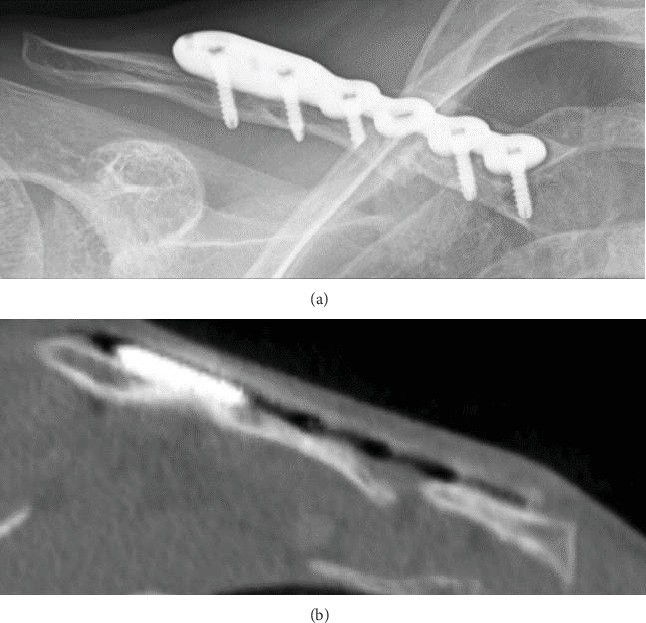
(a, b) Radiographs and computed tomography revealed nonunion and implant loosening one year after the initial surgery.
